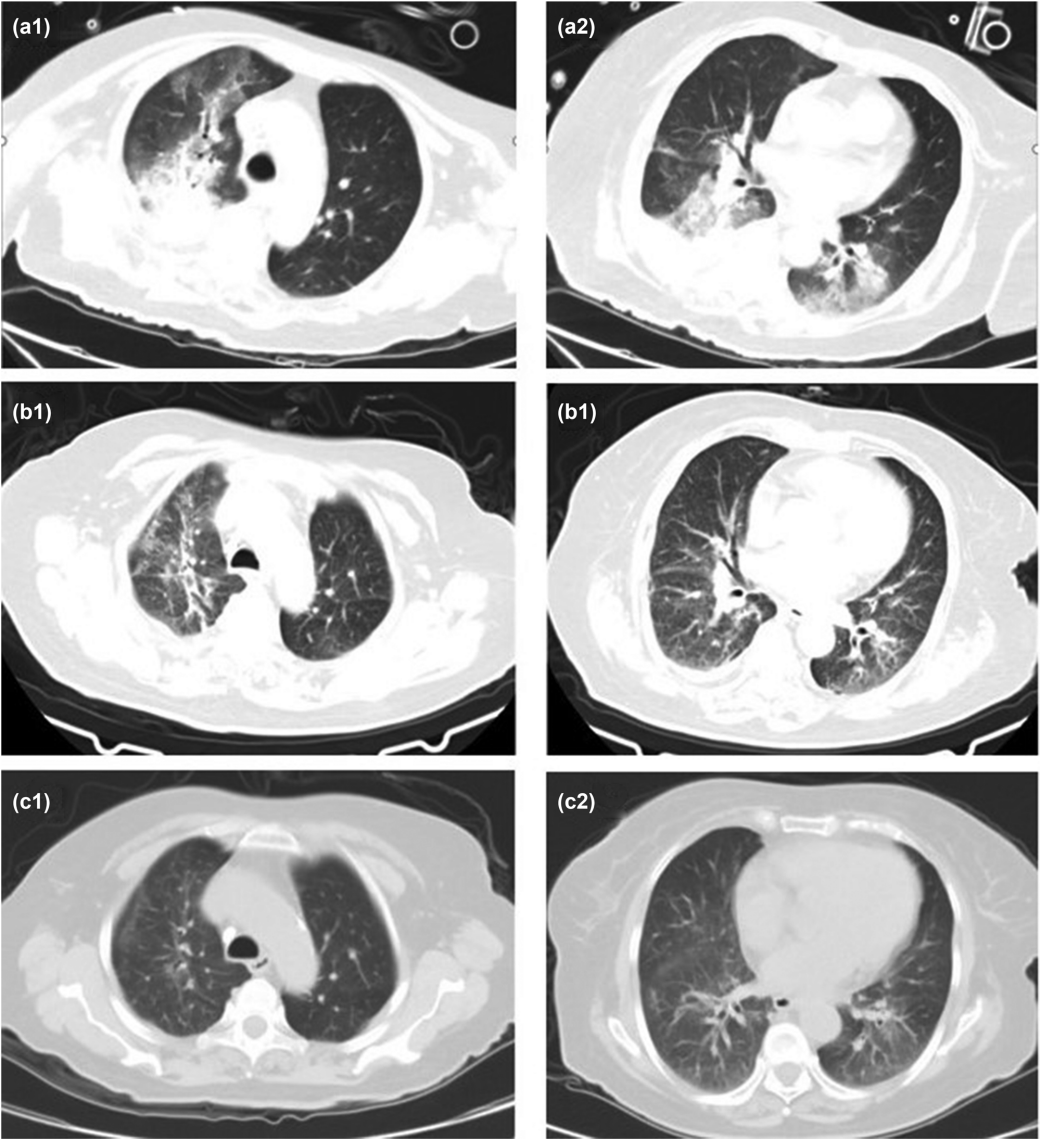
CT images (a1) and (a2) of case 7 (2021. 02.23) are admission D8, DOX D1, bilateral lung infection. (b1), (b2) (2021.03.04) are admission D18, DOX D11, and the bilateral lung infection lesions were slightly reduced. Pictures (c1) and (c2) (2021.03.27) were re-examined 2 weeks after discharge, and the bilateral lung lesions were significantly absorbed.
